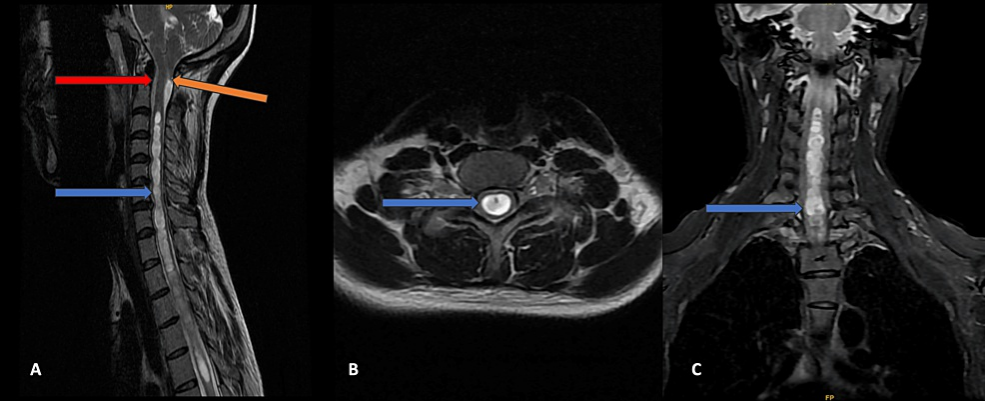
MRI of the cervical spine Magnetic resonance imaging (MRI) of the cervical spine, mid-sagittal T2-weighted image, shows tonsillar herniation (orange arrow), medullary kinking (red arrow), and hydrosyringomyelia (blue arrow) (A). Axial and coronal MRI T2-weighted images show hydrosyringomyelia (blue arrow) (B and C).
